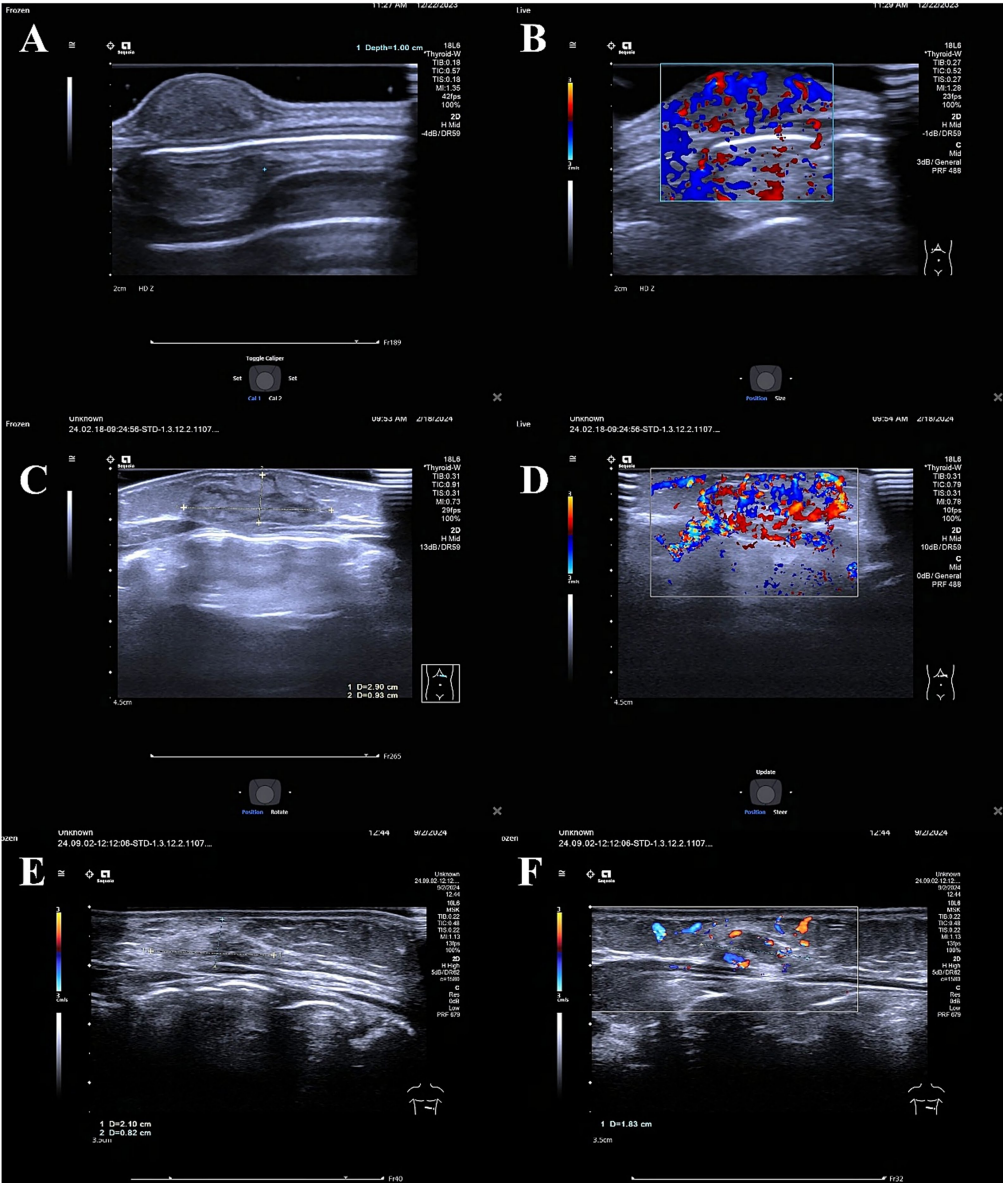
Ultrasound images of a hemangioma of the deep fascia of the abdominal wall in a 17-day-old female infant treated with bleomycin. (A,B) Pre-treatment, the tumor shows hypoechoic appearance with uniform echogenicity, clear margins, and abundant internal blood flow. (C,D) Two months after the first sclerotherapy, the tumor presents with slightly hypoechoic appearance, clear margins, heterogeneous echogenicity with a reticular pattern, and abundant internal blood flow. (E,F) Two months after the second sclerotherapy, the tumor shows slightly hyperechoic appearance, clear margins, heterogeneous echogenicity with a reticular pattern, and significantly reduced internal blood flow compared to before.
